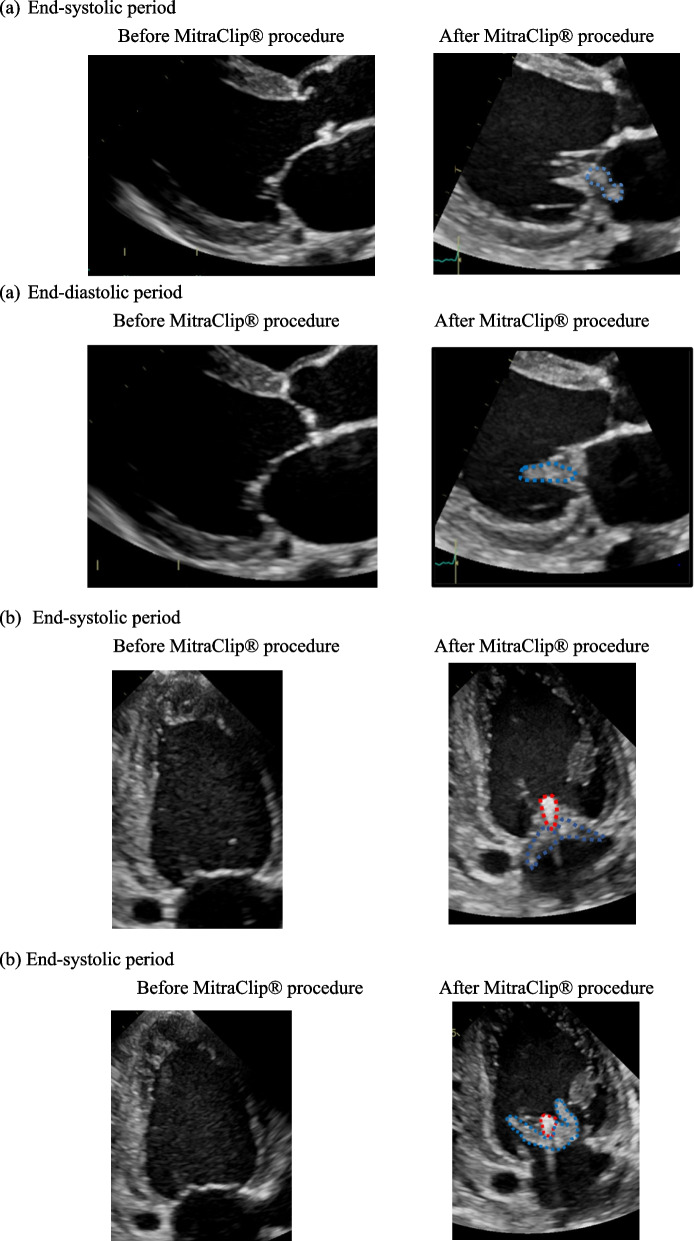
Giant vegetation that had adhered to the MitraClip® was observed when compared to before the MitraClip® procedure. The vegetation had extended to the medial site and lateral site of the native mitral valve and was mobile. The giant vegetation moved into the left ventricular cavity during the diastole period and into the left atrial cavity during the systole period. a Parasternal view. Blue dotted line: vegetation. b Two-chamber view. Red dotted line: MitraClip®. Blue dotted line: vegetation
